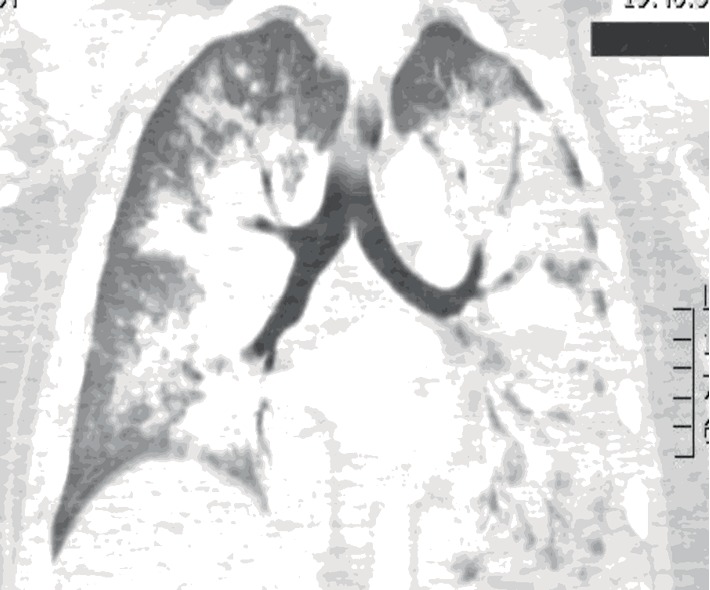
CT chest (coronal view) demonstrating bilateral diffuse lung infiltrates.
